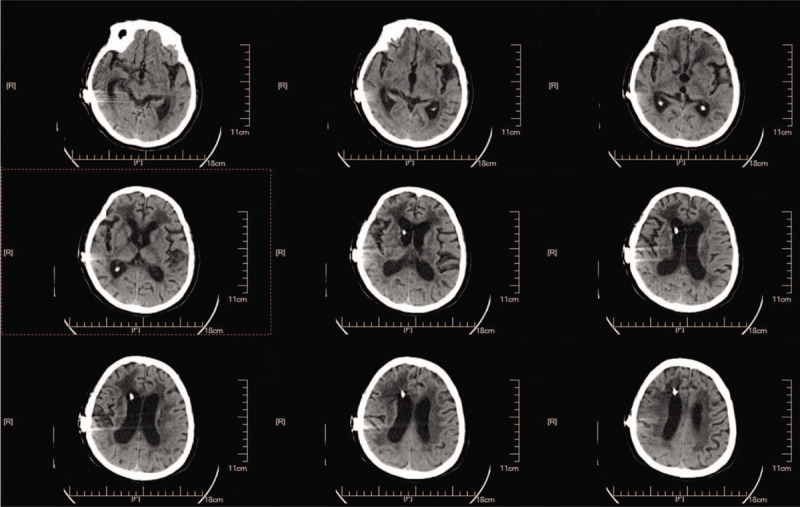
Preoperative abdominal X-ray scan performed during the second admission revealed the shunt tube to have been severely curled around the gallbladder.
